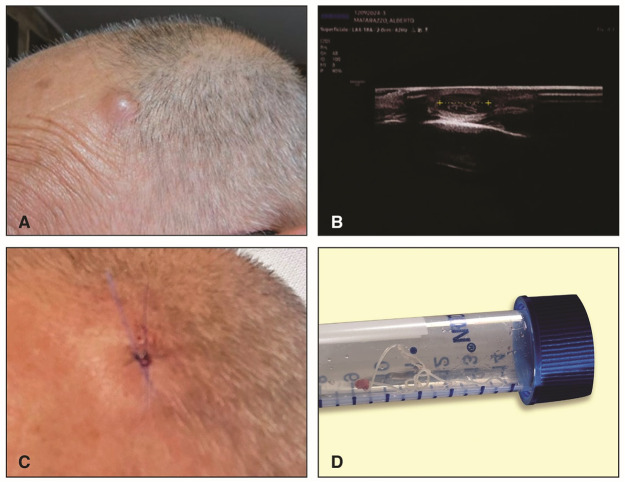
Case 2. (A) Patient with a subcutaneous mass located in the left frontal region of the scalp; (B) an ultrasound of the skin revealed an oval-shaped, hypoechoic nodule measuring 7.7 × 3.9 mm with well-defined, avascular margins in the scalp area, suggestive of the presence of a nematode; (C) patient after surgery; (D) a 10 cm-long nematode surgically removed from the nodule in the scalp area.
